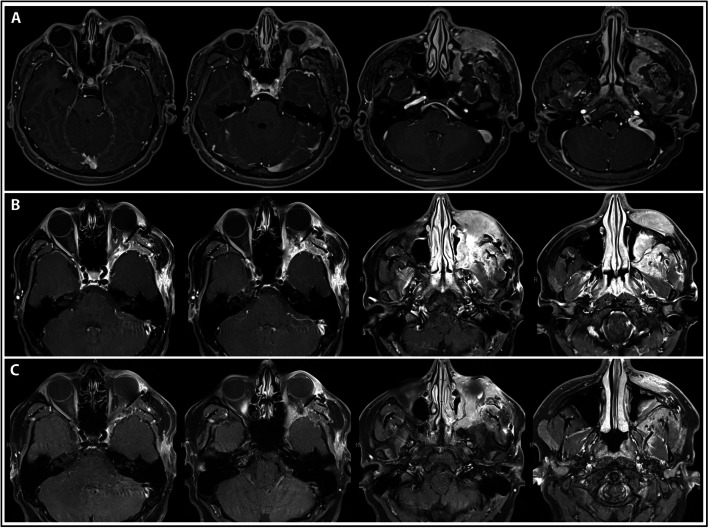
Postoperative and follow-up MRI brain and orbits after initial and second surgical debulkings. a Postoperative T1-weighted post-contrast MRI brain and orbits obtained after initial surgical debulking demonstrating significant resection at the level of the optic nerve with residual enhancing mass in the infraorbital region, left cheek, and maxillary sinus. There is residual circumferential enhancement around the optic nerve at the orbital apex. A small postoperative fluid collection along the lateral and inferior left orbit is also observed. b Follow-up T1-weighted post-contrast MRI obtained approximately 2 months after initial surgical debulking showing increased lobular enhancing soft tissue mass protruding through the left orbital floor into the left maxillary sinus. Increased enhancement in the left retromaxillary fat and along the left maxillary alveolar ridge are consistent with progressive disease. c Follow-up MRI obtained approximately 1 month after second surgical debulking demonstrating interval resection of the premaxillary and infraorbital soft tissue enhancement without evidence of disease progression
